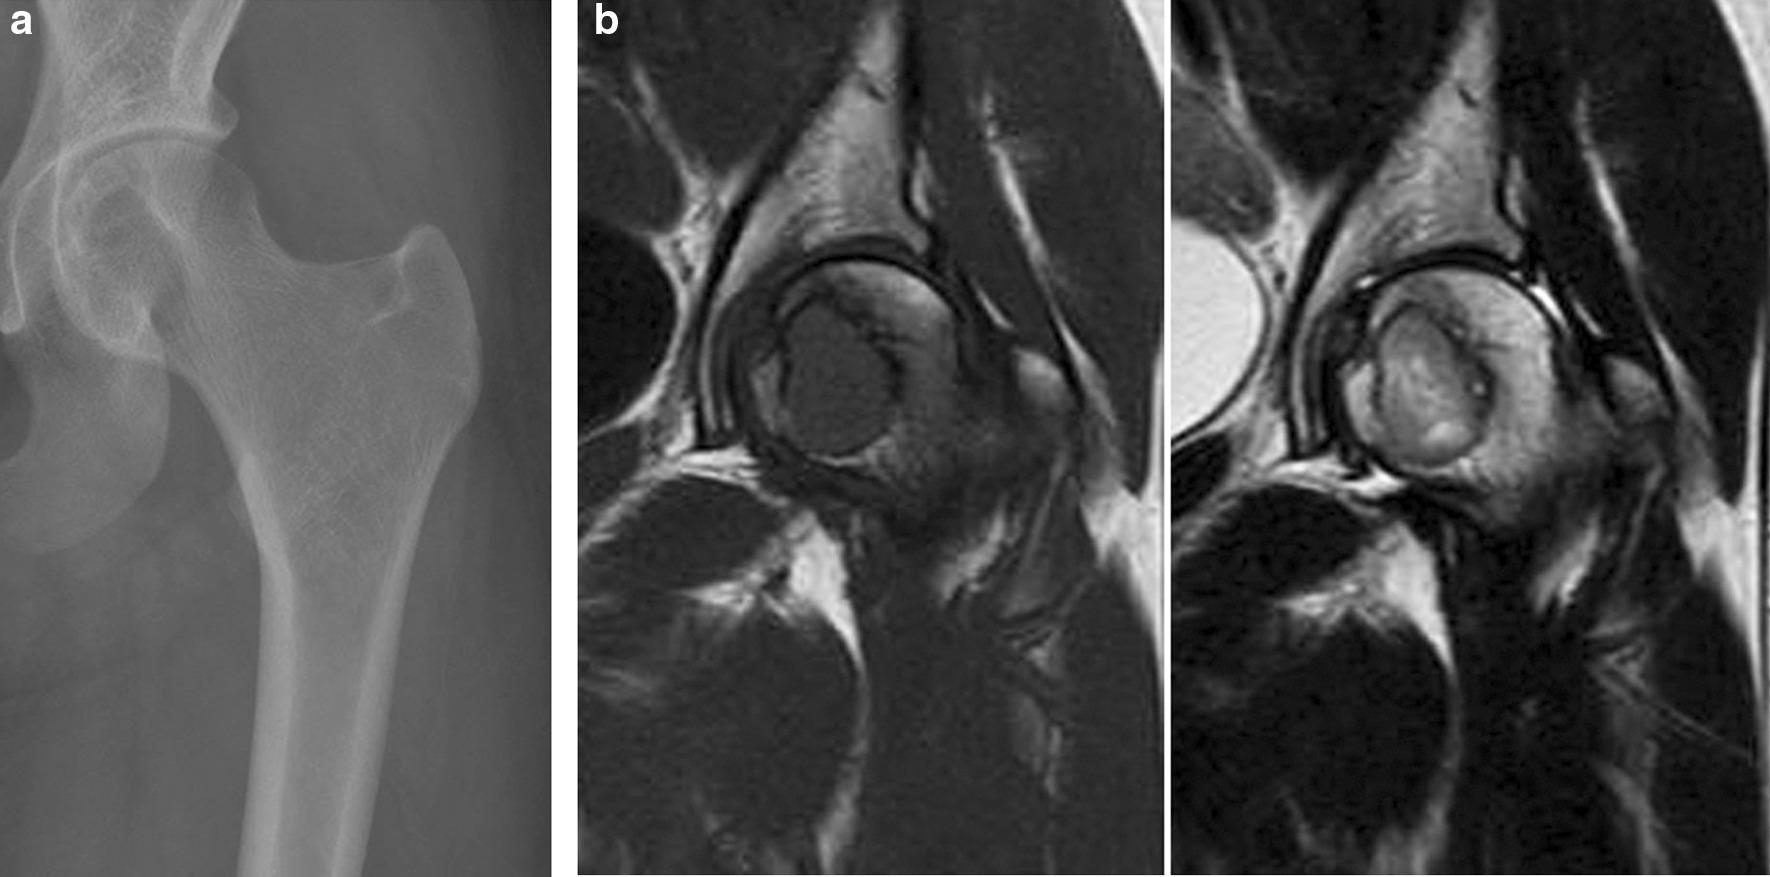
Preoperative images: Case 1. a X-ray image showing a 2 cm × 2 cm osteolytic lesion with a marginal sclerotic rim. b MRI revealing a lesion that is low intensity on T1 (left) and heterogeneously high intensity on T2 (right) imaging, with perilesional edema
